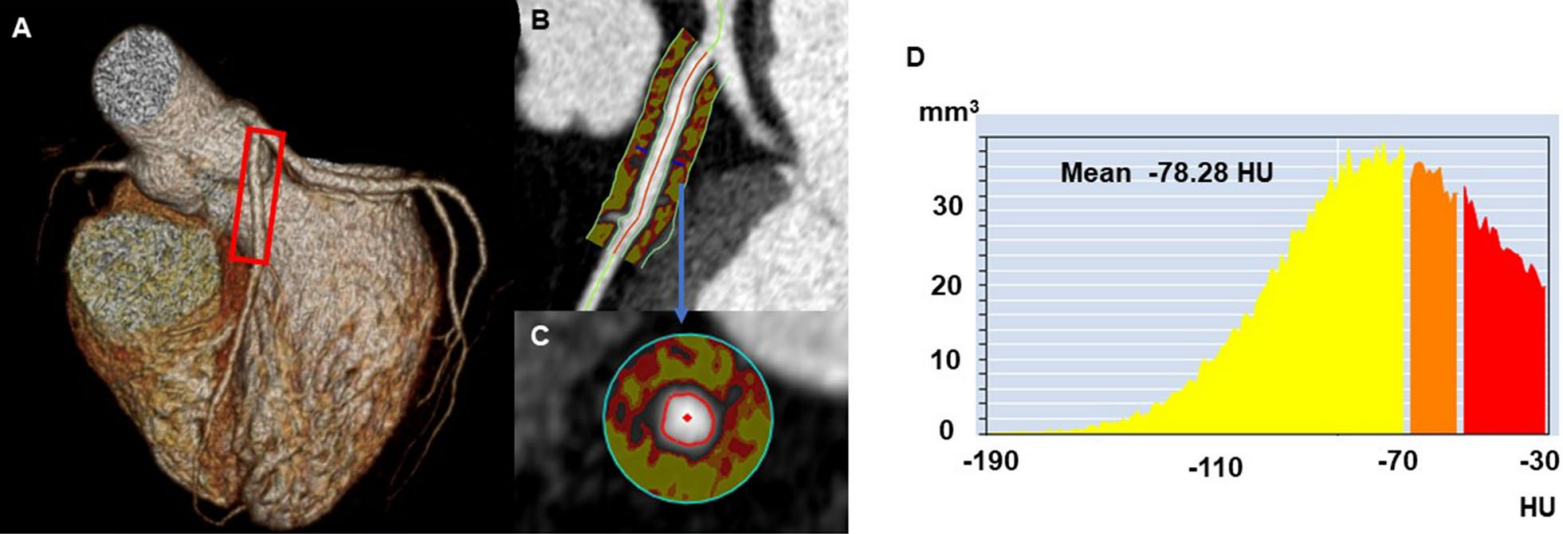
Representative case of pericoronary adipose tissue attenuation measured by coronary computed tomography (CT) angiography. Three-dimensional reconstruction of the heart (A); Pericoronary adipose tissue attenuation between − 190 and – 30 HU in the longitudinal view (B) and the cross-sectional view (C); around the proximal 40 mm of the left anterior descending artery; histogram of CT attenuation within the traced area (D)
